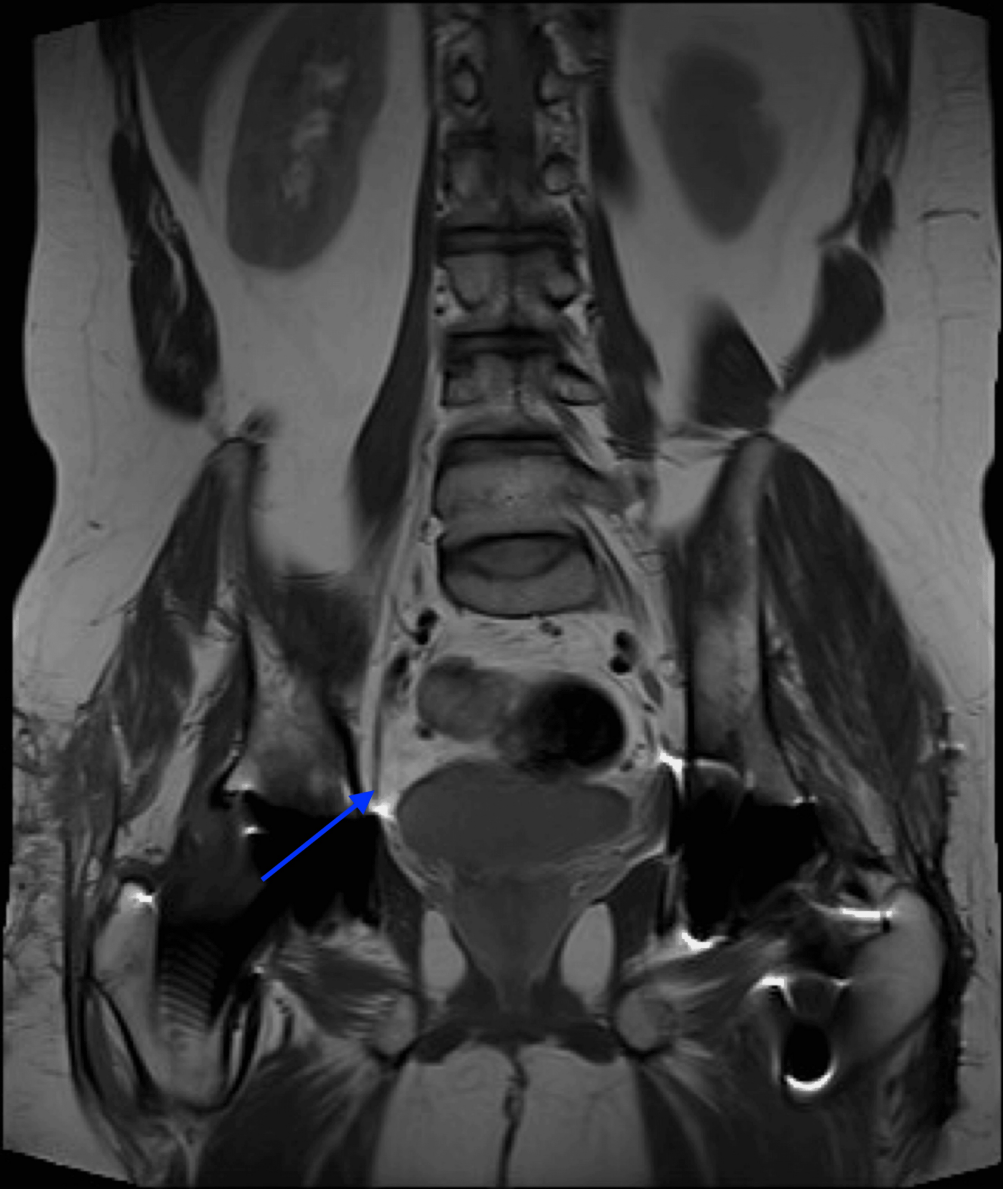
Magnetic resonance imaging showing an intact right femoral nerve along its course into the thigh
